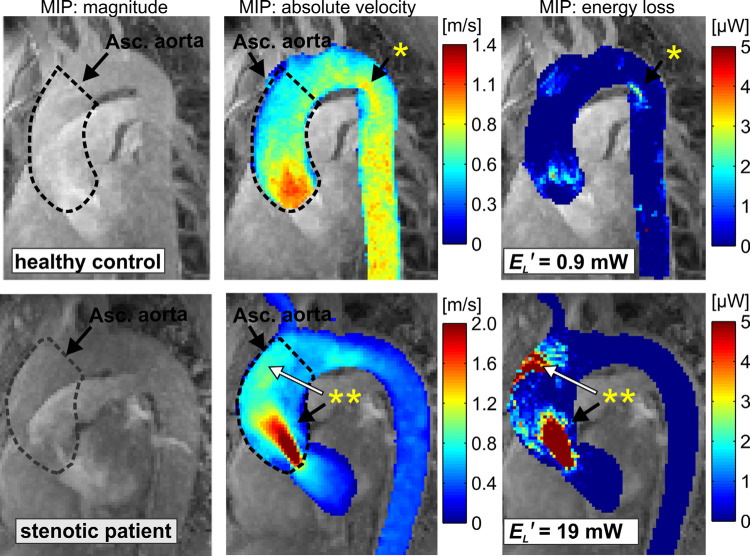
Four-dimensional (4-D) flow MRI images showing the velocity field and energy loss through the aortic valve and aorta. E_L_′, cumulative peak systolic energy loss in the ascending aorta. Adapted from Ref. 241, with permission from *Magnetic Resonance in Medicine*. Arrows, *, and  are from source image and have no significance in this review.
